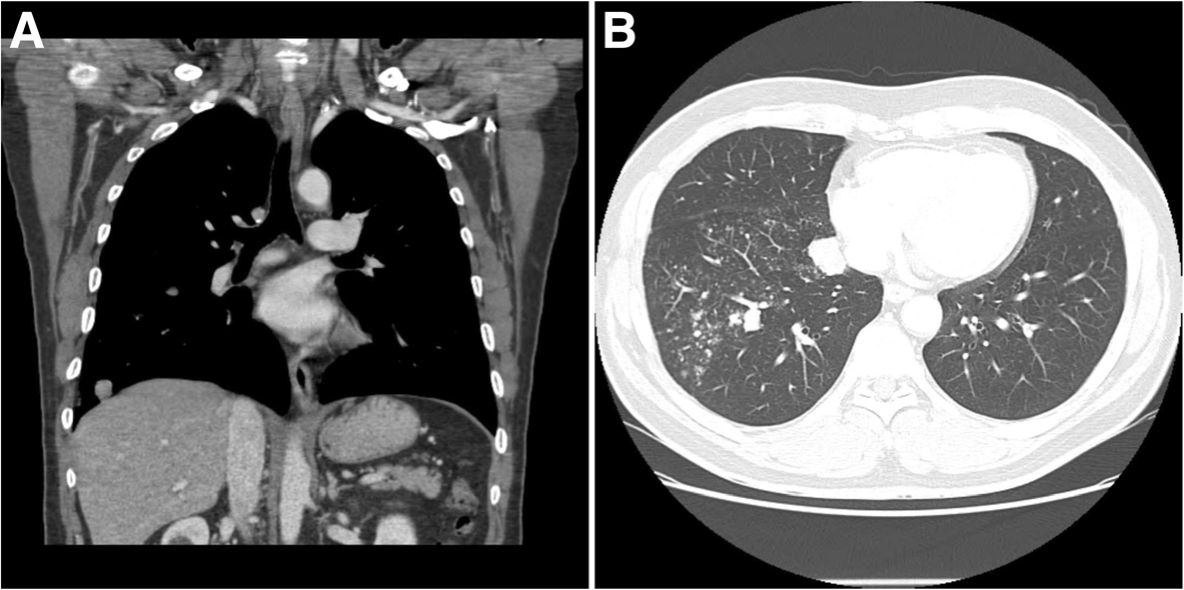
Preoperative chest computed tomography. (A, B) CT shows numerous nodules with some calcifications in the right middle and lower lobes.
